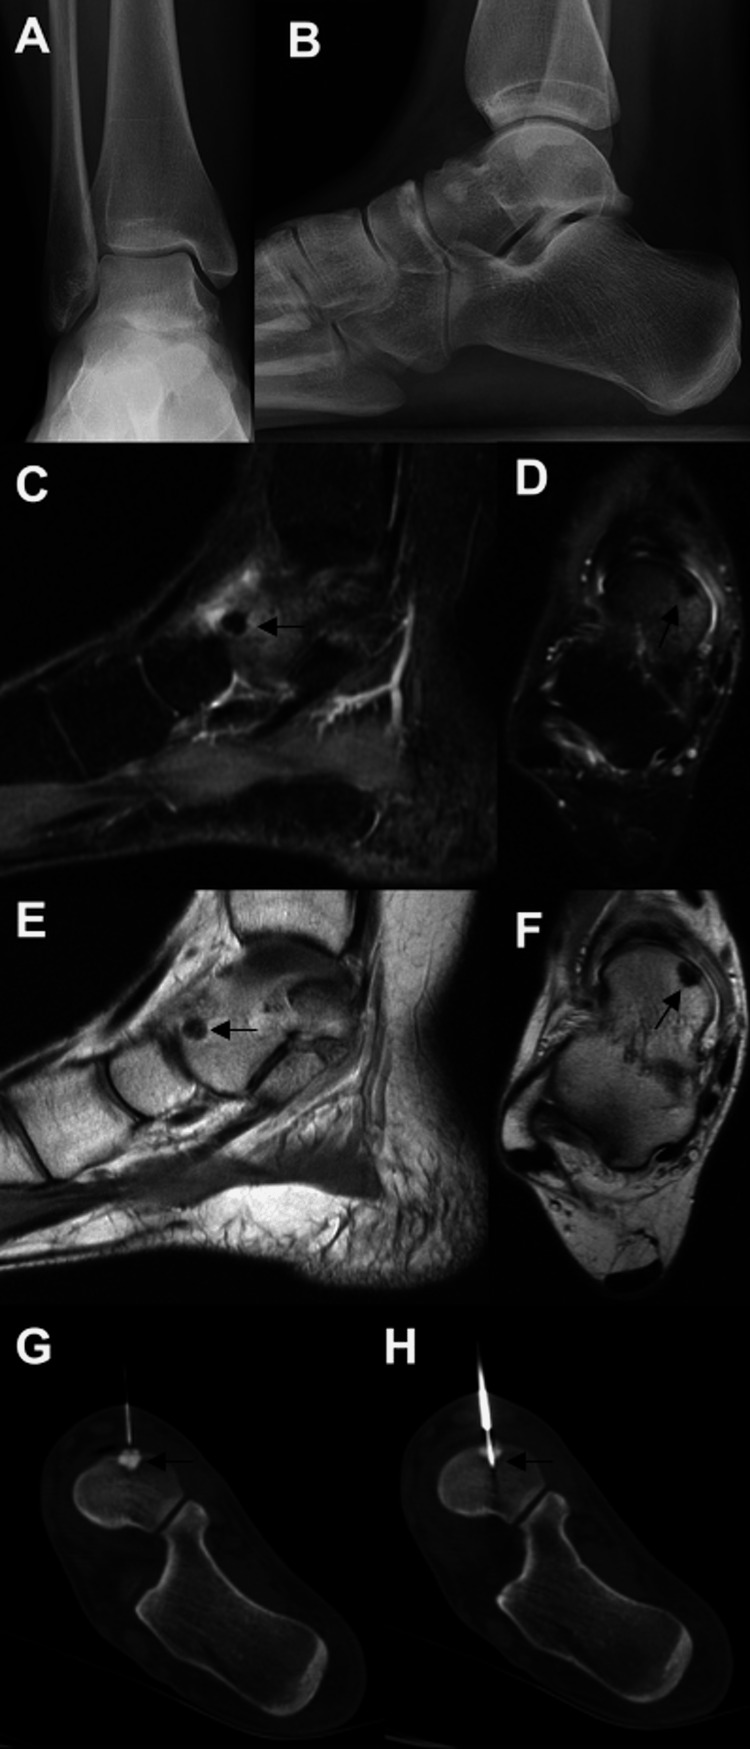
Images of the patient discussed in case three. Repeat radiographs of the ankle performed at our institution three months after initial presentation to outside health care provider (A, B) which showed a small sclerotic area in the anterior talus. MRI (sagittal (C) and axial (D) inversion recovery, and sagittal (E) and axial (F) proton density) showed a low-signal area with surrounding edema, consistent with osteoid osteoma. RFA was successfully performed on the lesion under CT guidance (G, H). MRI: magnetic resonance imaging; CT: computed tomography; RFA: radio-frequency ablation
